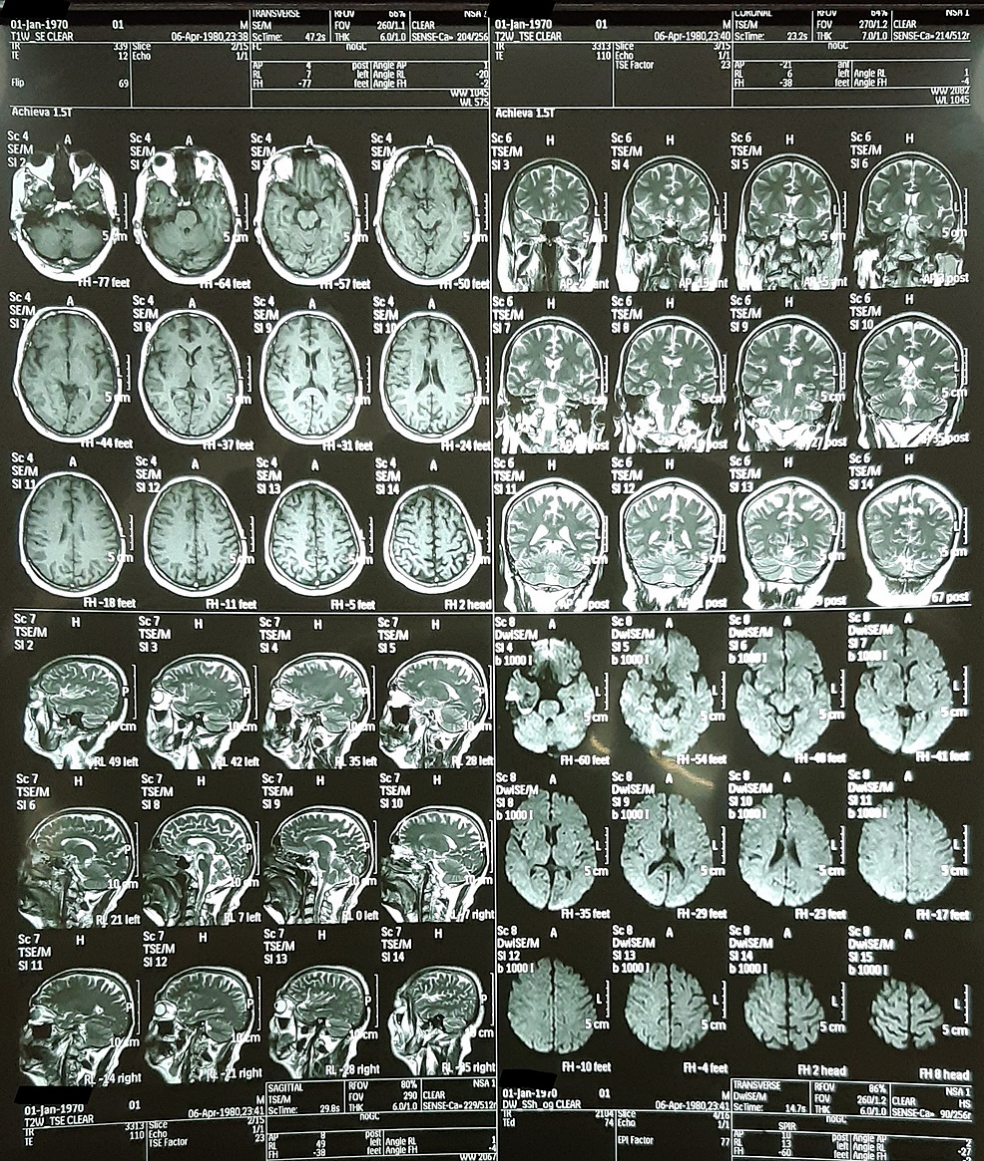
Showing sagittal sections of a T1 weighted MRI- No abnormal findings noted.
